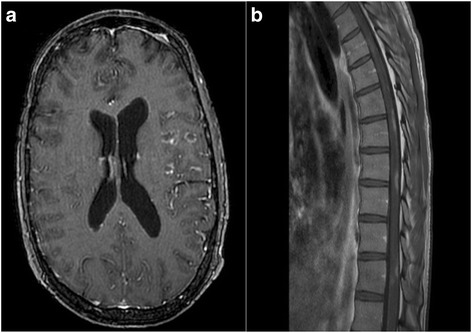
Magnetic Resonance Imaging (MRI) of the brain demonstrated multiple enhancing lesions involving the cerebellum, left midbrain, left pons. A right medullary lesion was also visualized in addition to multiple foci of enhancement involving the cerebral hemispheres (a). MRI of the spinal cord showed multiple T2 hyperintensities within the thoracic spine with associated swelling. Faint leptomeningeal enhancement at the anterior cervical cord was also visualized (b)
